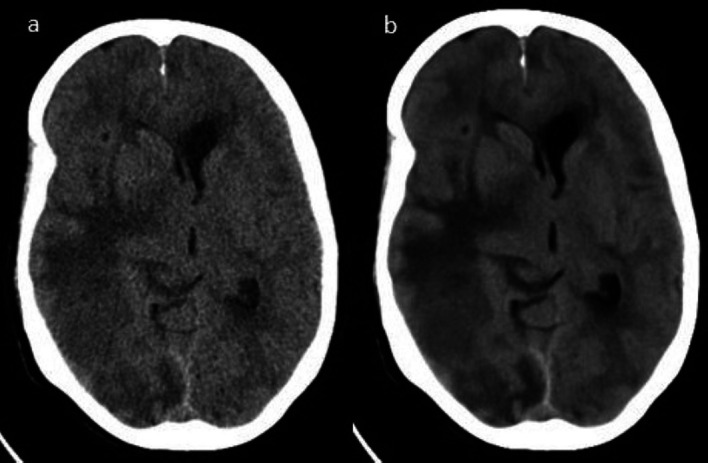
Axial CT images showing the improved image quality with (a) iDose
